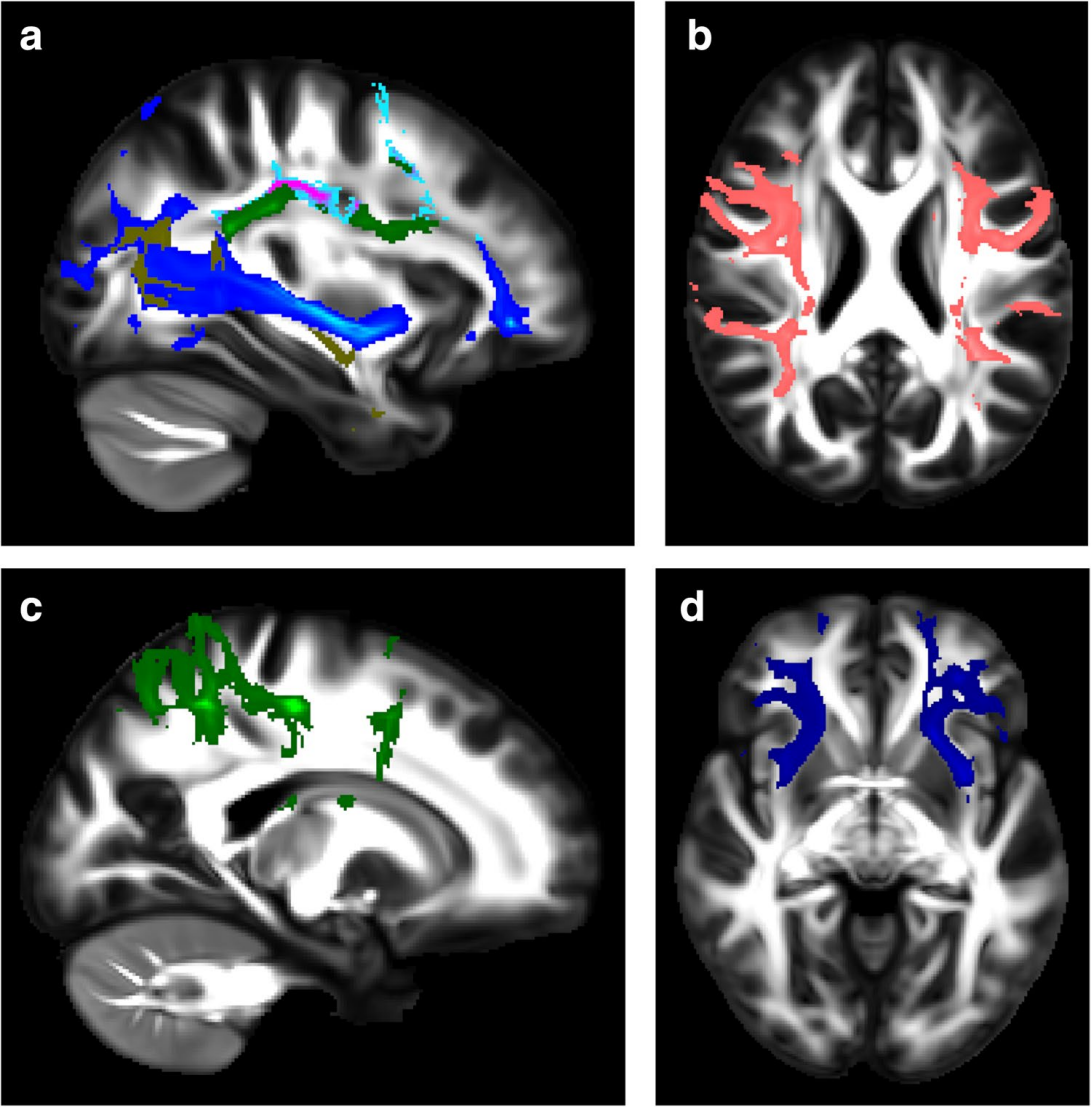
T1-weighted images with an overlay of the language-related tracts coded by colours in a 9.1-year-old healthy control girl who had normal findings on conventional magnetic resonance imaging. a A left sagittal image of the superior longitudinal fasciculus 2 is displayed in turquoise/pink, the superior longitudinal fasciculus 3 in green, the inferior fronto-occipital fasciculus in blue/light blue and the inferior longitudinal fasciculus in dark yellow. b An axial image with the left and right arcuate fasciculi displayed in red. c A left sagittal image with the superior longitudinal fasciculus 1 displayed in green. d An axial image with the left and right uncinate fasciculi displayed in blue
